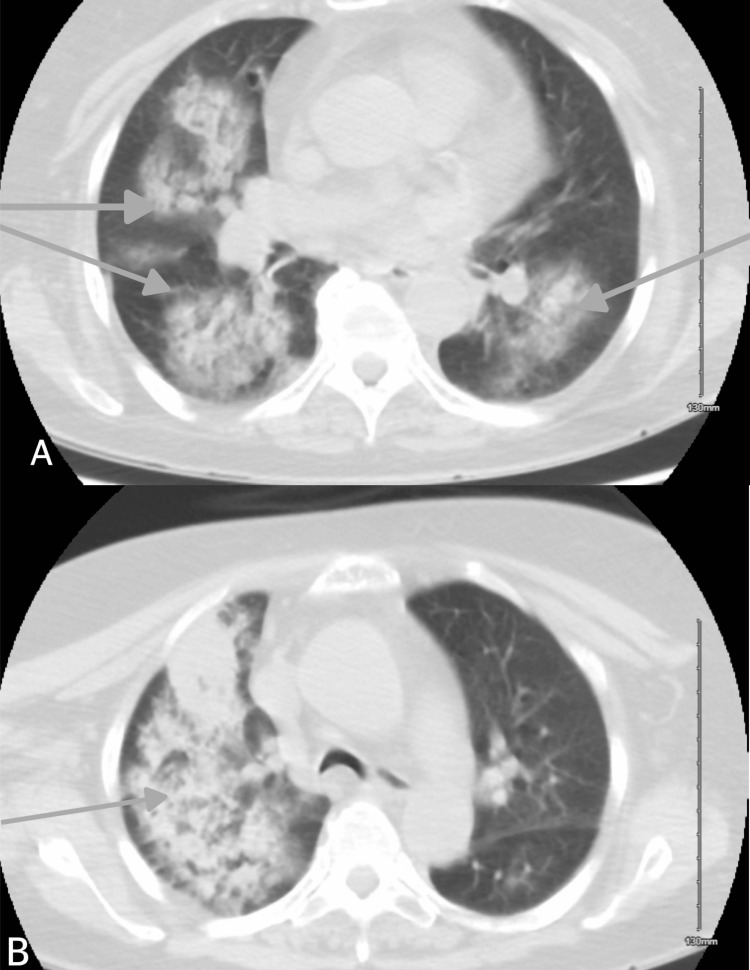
Axial view, lung window, of repeat computed tomography scan showing progression of multilobar infiltrates (gray arrows) A) Axial CT of the chest at the lower lobes/bases, showing bilateral patchy airspace opacities with a mix of ground-glass and consolidation changes. The opacities are multifocal and peripheral. B) Axial CT of the chest showing predominantly right upper lobe confluent patchy consolidation with surrounding ground-glass opacities, with the left upper lobe relatively well aerated. CT: computed tomography
